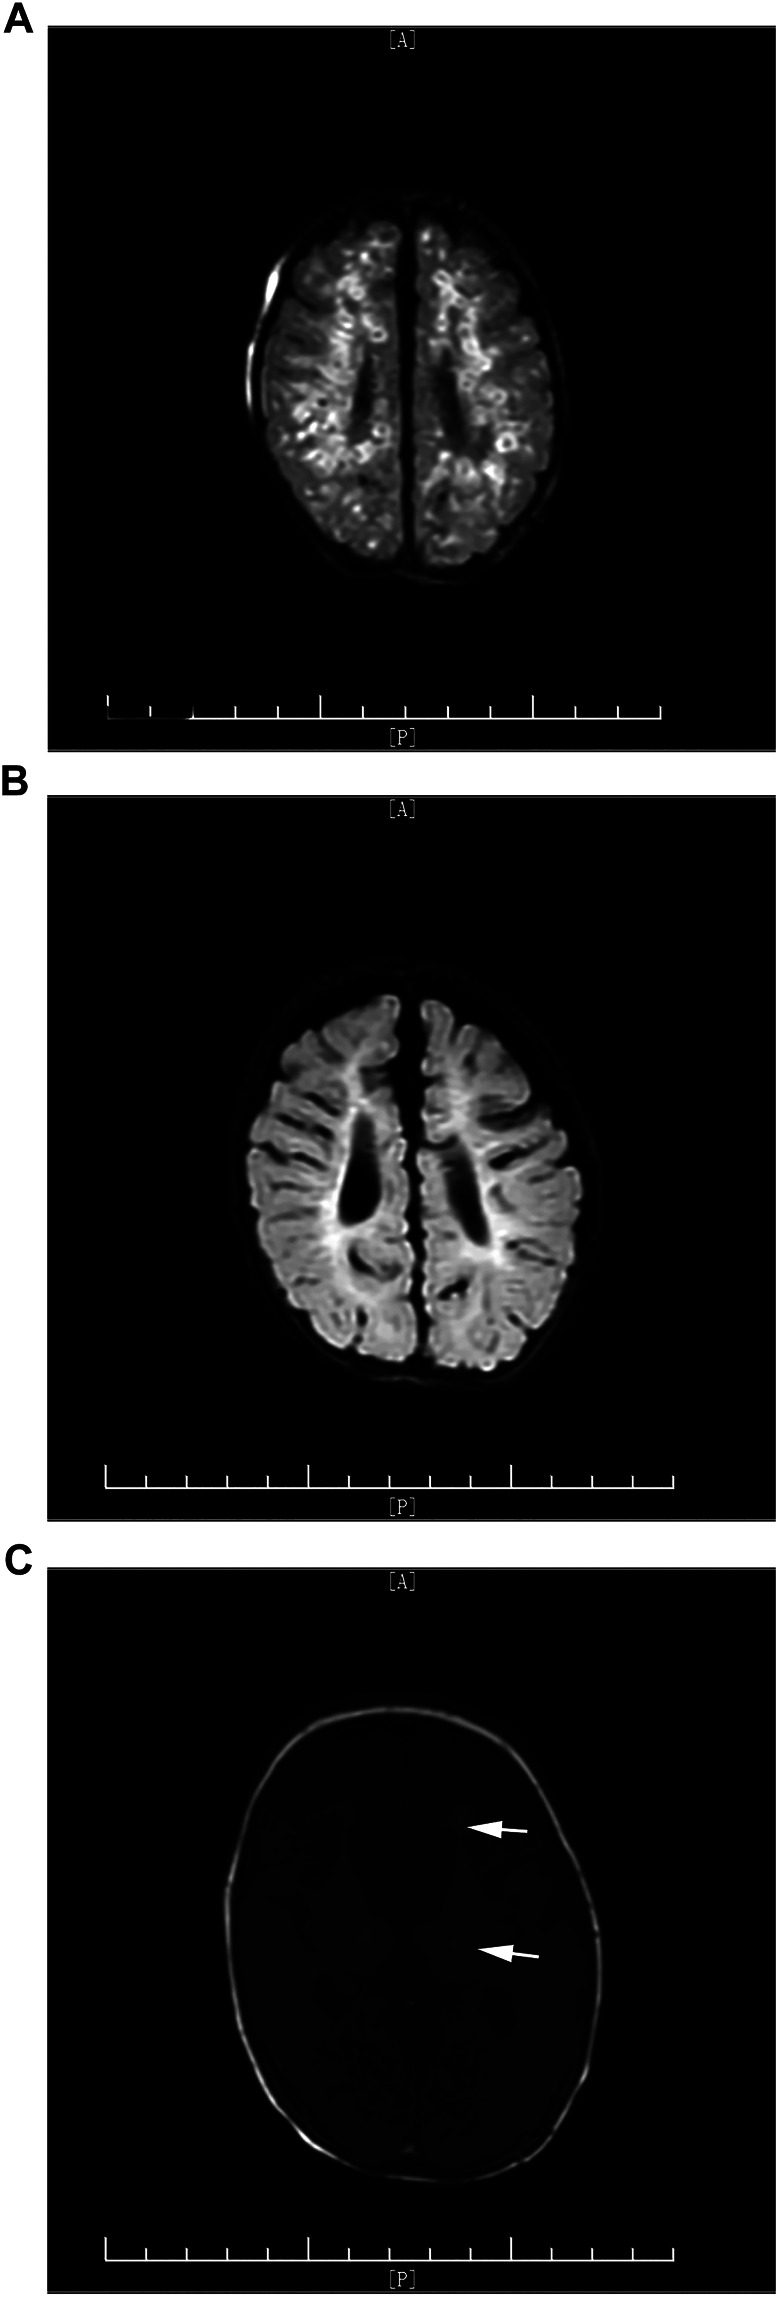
(A) Multiple nodular and circle-enhanced signals in T2-FLAIR at 1 week after infection. (B) Clearance of enhanced signals before treatment was discontinued. (C) Arrows indicate paraventricular white matter injury in T1WI before treatment was discontinued.
